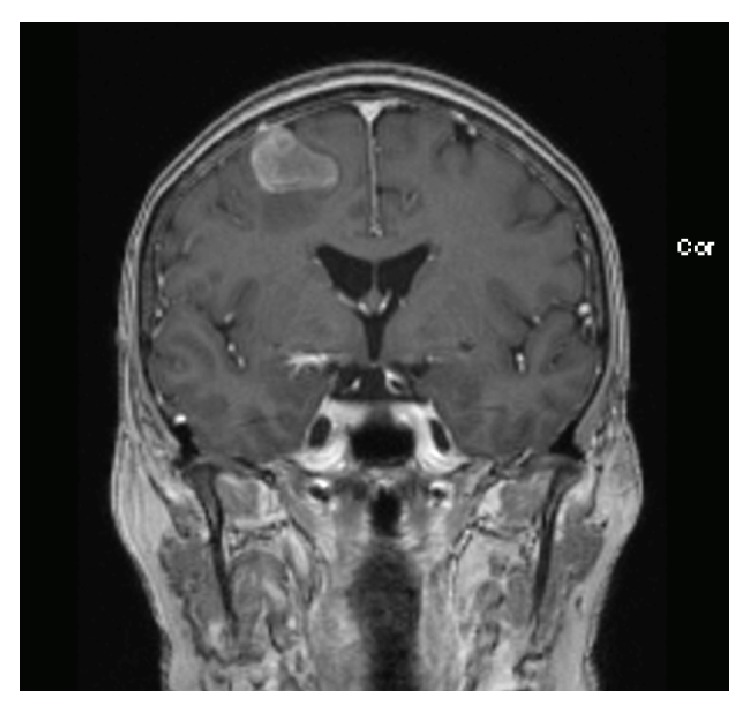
2014 MR image, coronal view.
